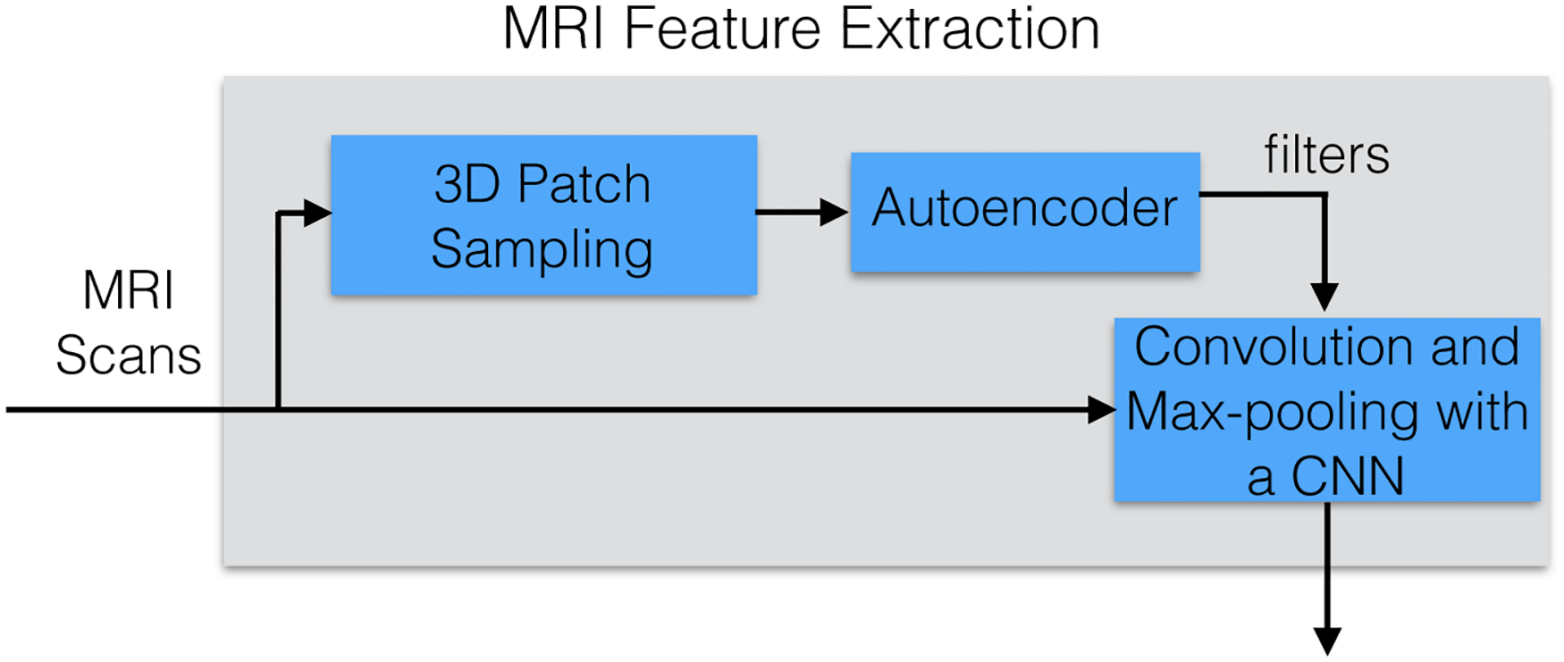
Process of extracting MRI features to be provided to a learning algorithm. This expands the “MRI feature extraction” box from Fig 1.
